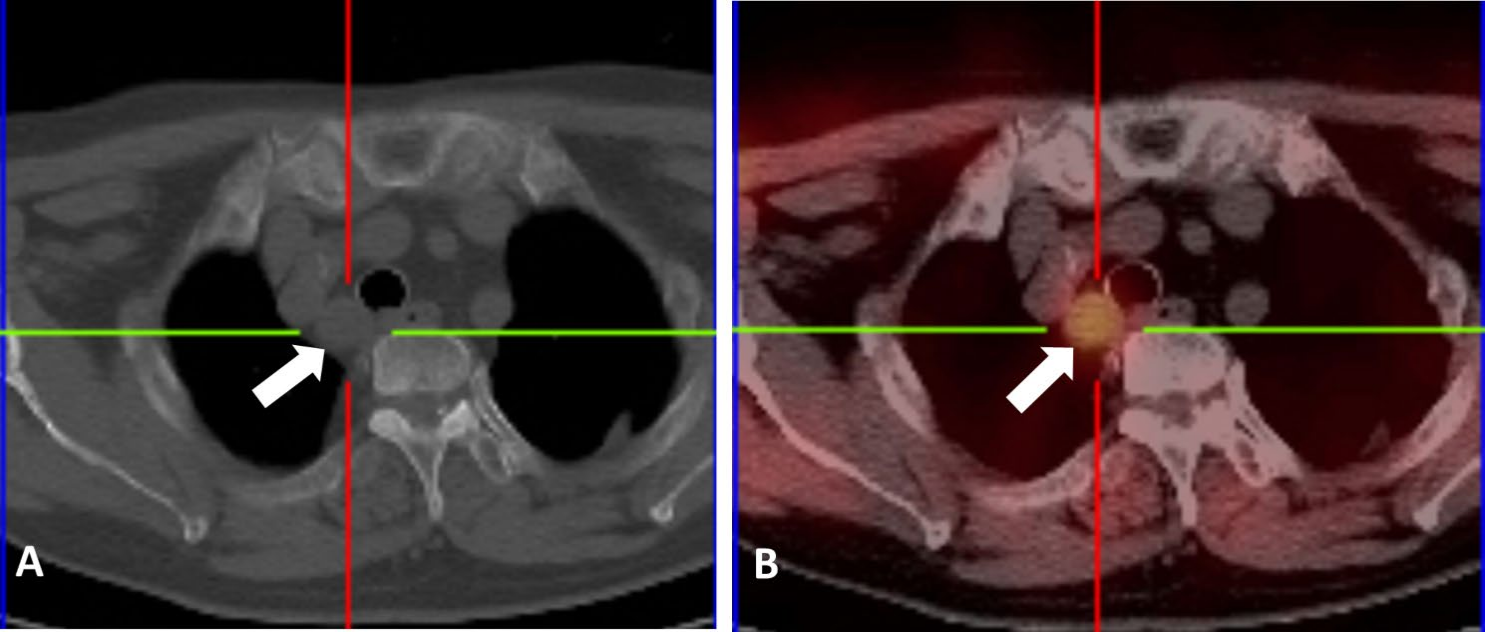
Patient imaging. (A) Contrast-enhanced computed tomography of the chest demonstrating a 2.4x1.4cm solid posterior mediastinal mass with contrast enhanced in the edge abutting the trachea and esophagus(arrow). (B) 99mTechnetiummethoxy-isobutyl-isonitrite (MIBI) scintigraphy demonstrating a large distinct accumulation of radiotracer in the right posterior superior mediastinum(arrow)
